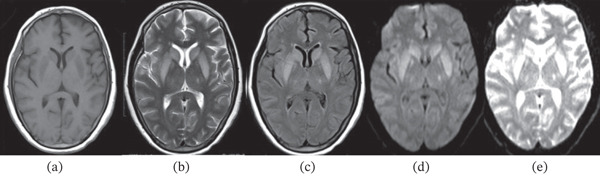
(a–e) T1, T2, FLAIR, DWI, and ADC images of the basal ganglia lesion demonstrating hypointensity on T1, hyperintensity on T2 and FLAIR, and T2 shine‐through.
